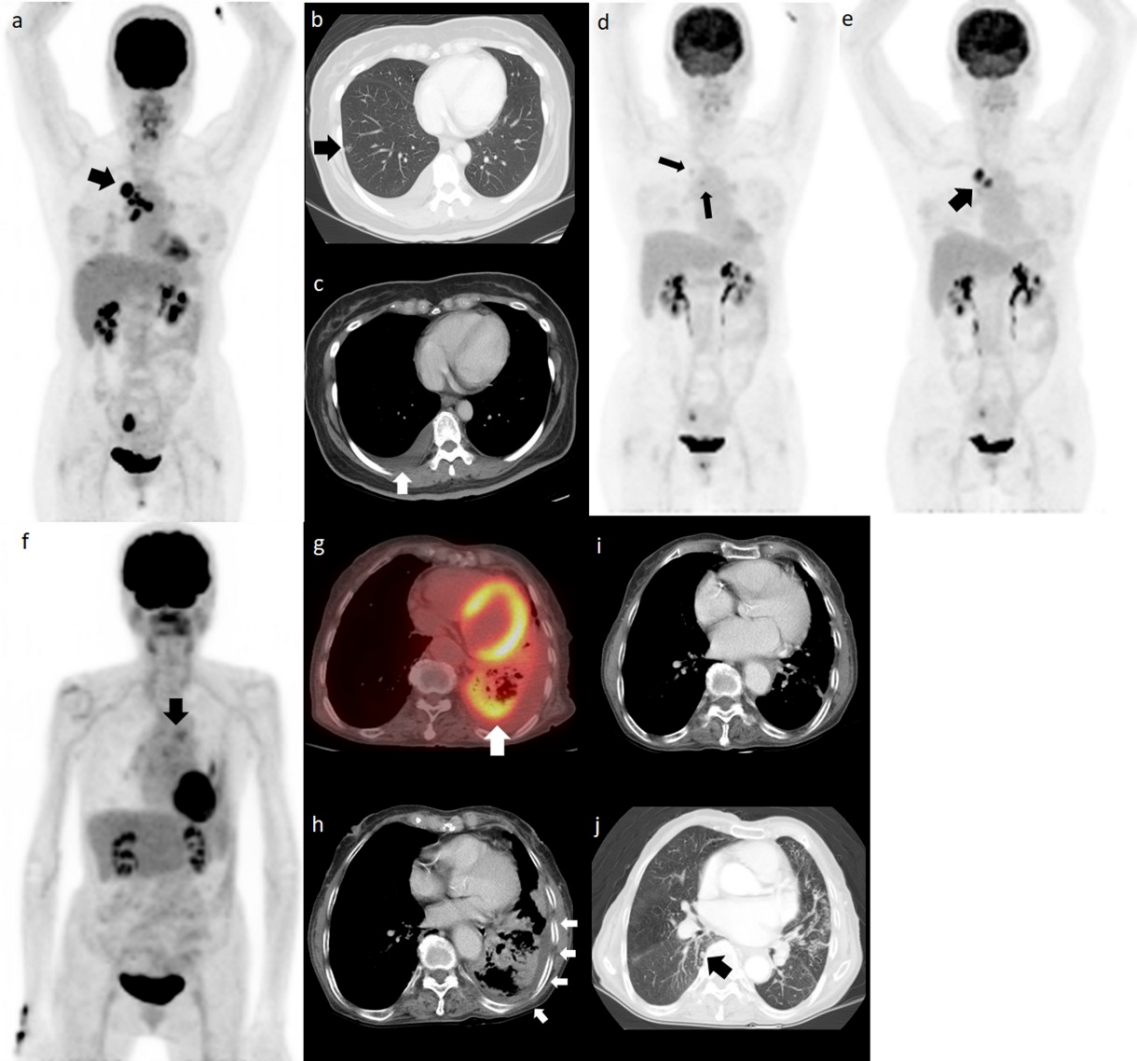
The survival prediction model outperforms the TNM staging system. Pretreatment ^18^F-FDG PET MIP image (a) for a 58-year-old woman with a primary tumor in the right upper lobe. Because of the presence of tiny nodule in different ipsilateral lobe and malignant pleural effusion (b and c, arrow), the clinical staging was cT4N3M1a. The EGFR mutation was L858R. The SUV entropy of the primary tumor was 5.32 (0.04 lower than the cut-off). A score of 1 was assigned for this patient. After 1 year of erlotinib treatment, the ^18^F-FDG PET MIP image (d) showed remarkable resolution of the primary tumor and metastatic lymph node ^18^F-FDG uptake (arrows). The patient experienced disease progression after 29.8 months of first line erlotinib treatment (e, arrow) and the treatment changed to osimertinib thereafter. The patient died of lung cancer with a PFS and OS of 29.8 and 54.3 months, respectively. The pretreatment ^18^F-FDG PET/CT and MIP images from an 83-year-old woman revealed mediastinal lymph node metastases (f, arrow) and an ^18^F-FDG avid tumor in the left lower lung (g, arrow). The patient also demonstrated malignant pleural effusion (h, arrows). Her clinical staging was cT3N3M1a and she had a deletion 19 EGFR mutation. The primary tumor SUV entropy was 5.44 (0.08 higher than the cut-off) and a score of 2 was given for this patient. She received erlotinib as a first line therapy. The CT acquired at 6 months after erlotinib treatment (i) showed resolution of the left pleural fluid; however, a new pulmonary nodule developed in the contralateral lung after 9.8 months of erlotinib treatment (j, arrow). The patient died of lung cancer progression with an OS of 18.1 months. MIP: Maximum intensity projection, PET: Positron emission tomography, EGFR: Epidermal growth factor receptor, PFS: Progression-free survival, OS: Overall survival.
